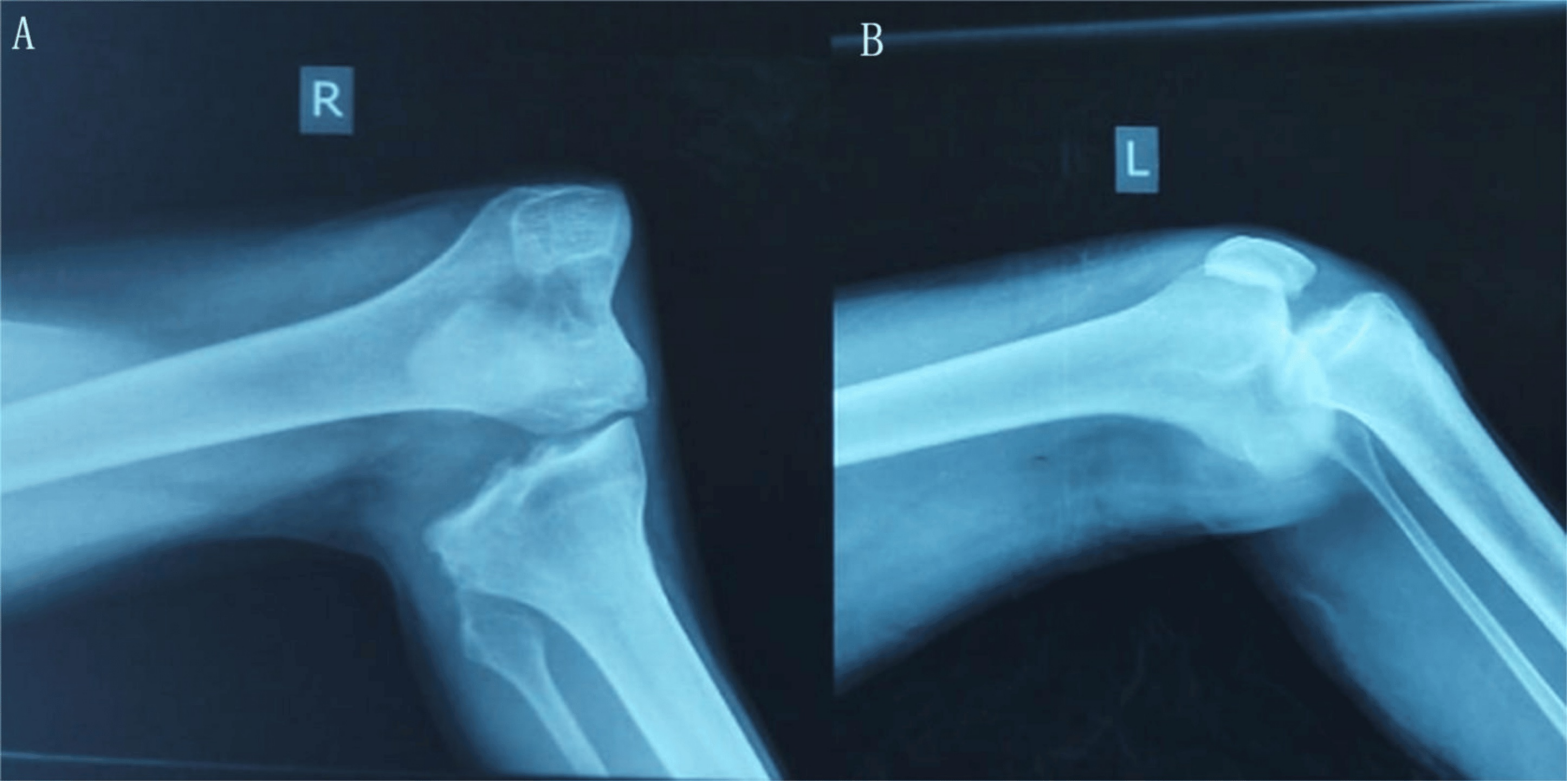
Bilateral knee dislocation on lateral radiographs Lateral radiographs of the right knee (A) and left knee (B) on admission, showing bilateral knee dislocation with complete loss of femorotibial congruence
